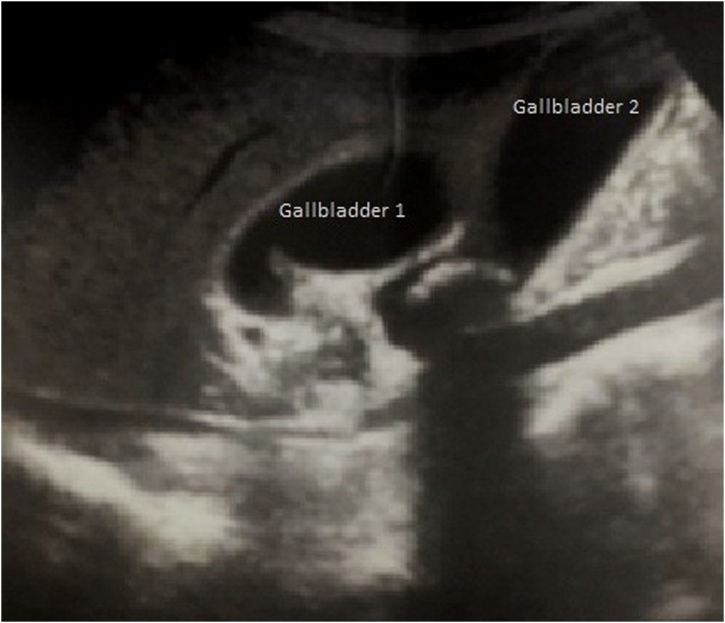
Ultrasonography findings showing two separate gallbladders (Gallbladder A and Gallbladder B) in fossa area.
